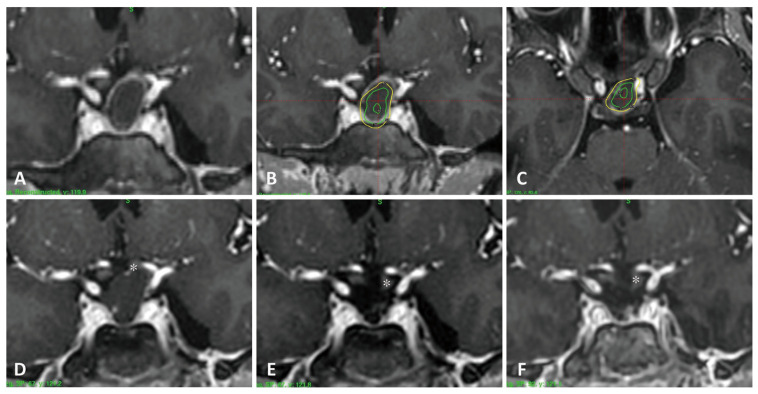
(A) Coronal preradiosurgical MRI showing tumor compressing the left optic nerve. (B,C) Coronal (B) and axial (C) dose-planning images. (D–F) Follow-up MRIs at 6 months (D), 12 months (E), and 24 months (F) demonstrate marked tumor shrinkage and decompression of the left optic nerve (white asterisk).
